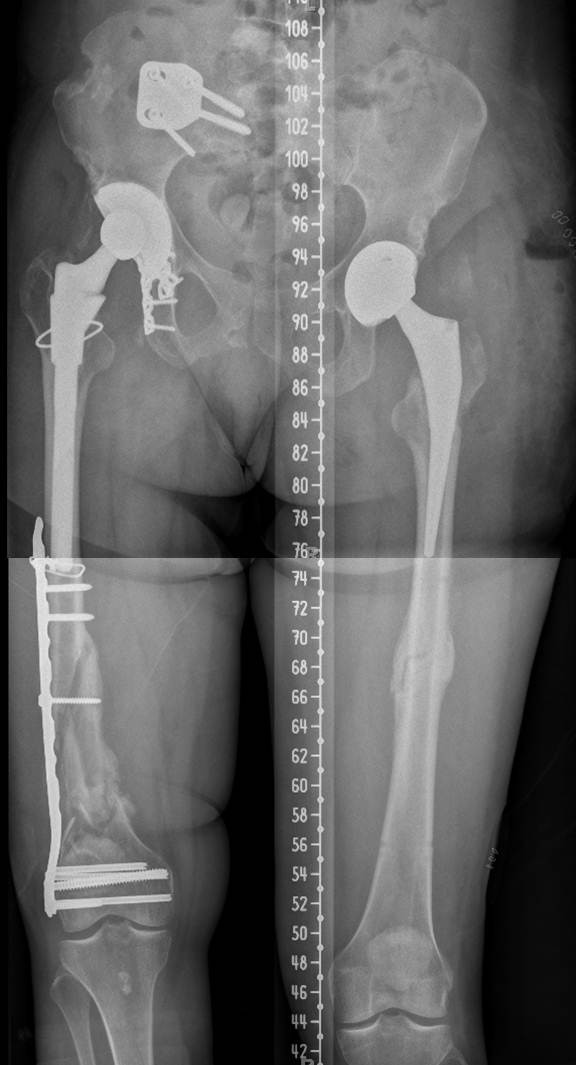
Full-length standing radiographs of bilateral femurs demonstrating a postoperative limb length discrepancy of 3.5 cm lengthening on the left side.
